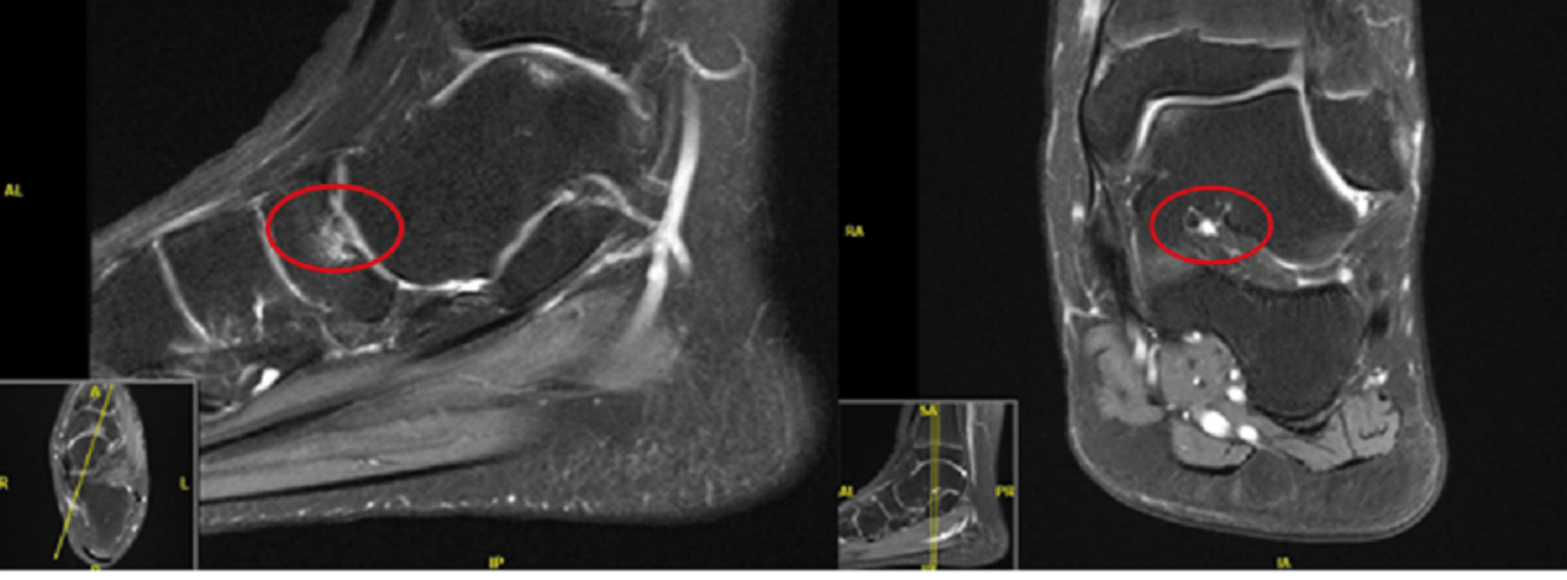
Left ankle repeat magnetic resonance imaging showing improving bony edema around the OCD lesion with no other abnormalities (red circles). OCD: osteochondritis dissecans
